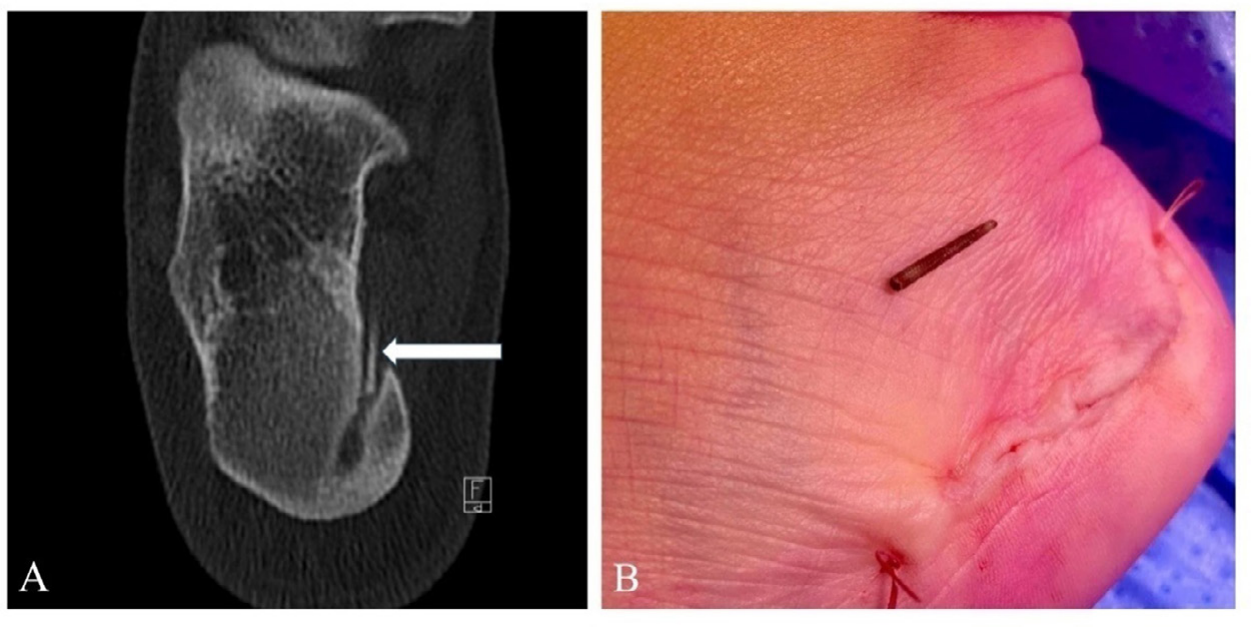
(A) CT scan demonstrates a linear structure within the abscess cavity extending into the soft tissues. (B) Intraoperative findings confirmed the presence of a coral fragment.
